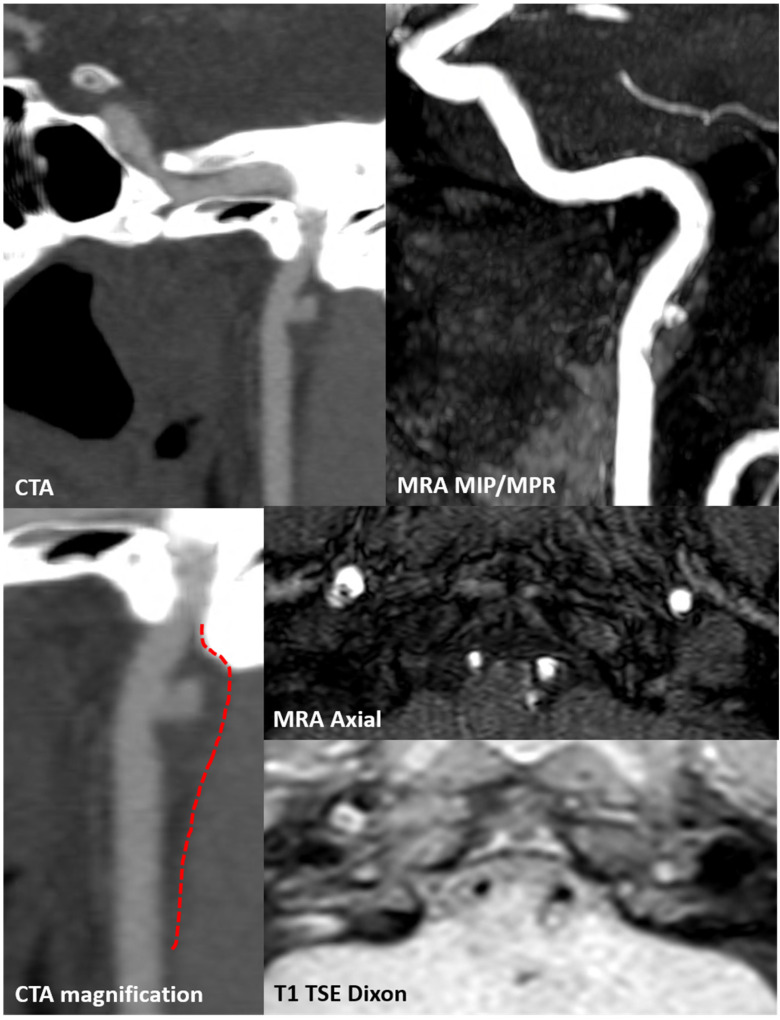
CTA and MRI study at 1 year from the first neuroimaging investigation. Both CTA and TOF MRA (MIP/MPR reconstruction on an oblique plane) showed a persisting atheroma with surface ulceration of the prepetrous segment on the right ICA. In the magnified CTA detail, the outer layer of the atheroma is outlined by a dotted red line and the positive remodeling is well evident.
